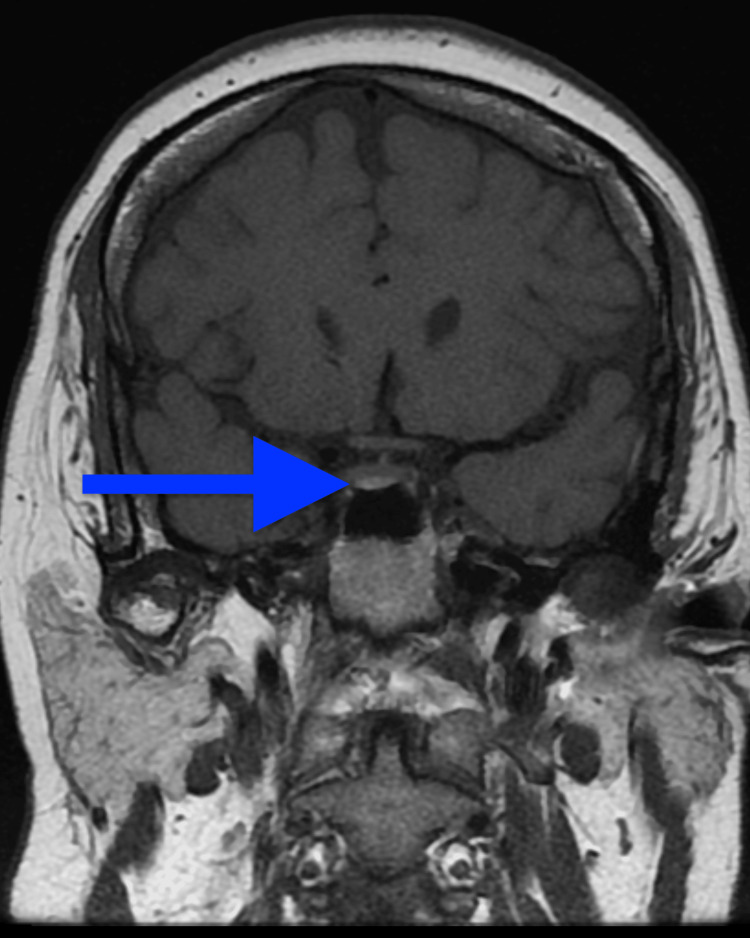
Pre-contrast MRI - sagittal section showing microadenoma (blue arrow).
